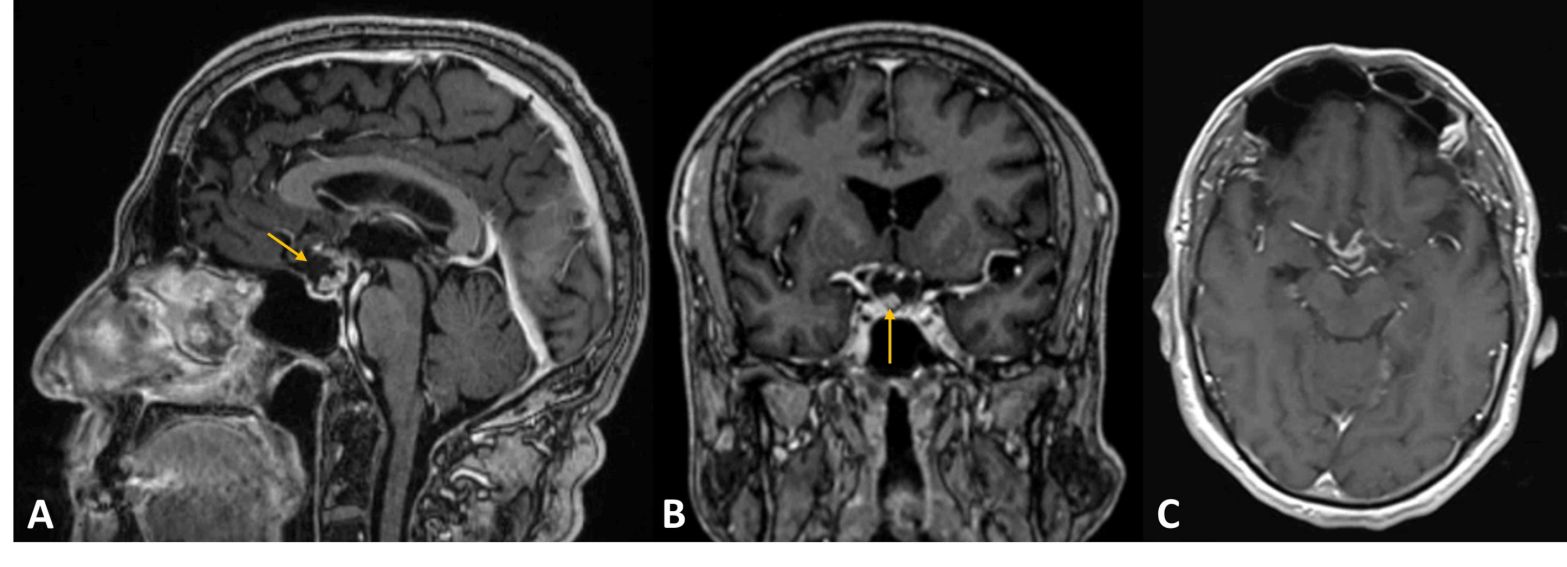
Preoperative MRI Sellar/parasellar extra-axial lesion with solid and cystic components. Contrast enhancement of the solid element and cystic wall. Gadolinium-enhanced T1-weighted images: (A) sagittal, (B) coronal, and (C) axial. Yellow arrows indicating the lesion.
